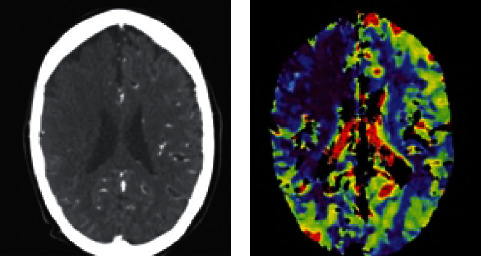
The (a) CT and (b) CTP images of ACI.
